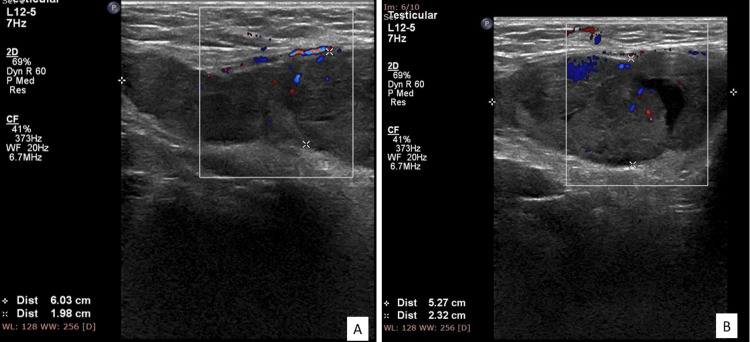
Ultrasound imaging of the scrotum Scrotal ultrasound showed a relatively well-defined hypoechoic lesion with internal cystic spaces measuring 6.0 x 1.9cm with areas of internal vascularity within the right inguinal canal region along the spermatic cord.
